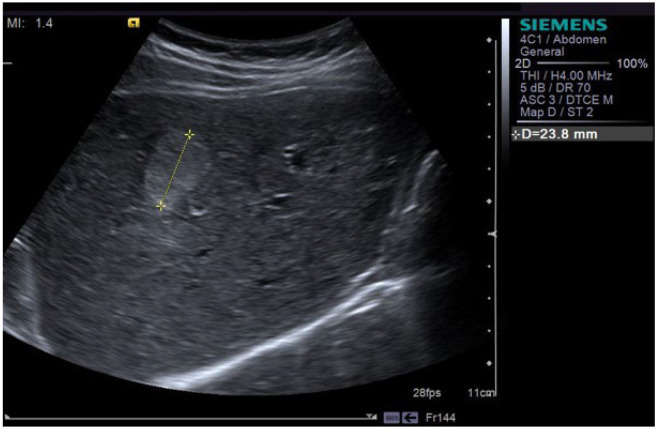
A 32-year-old male presents for consultation for occasional epigastric pain. Ultrasound revealed a hyperechoic, homogeneous, well delineated lesion (between markers +) 23 mm in diameter, situated in segment V—aspect of typical hemangioma.
